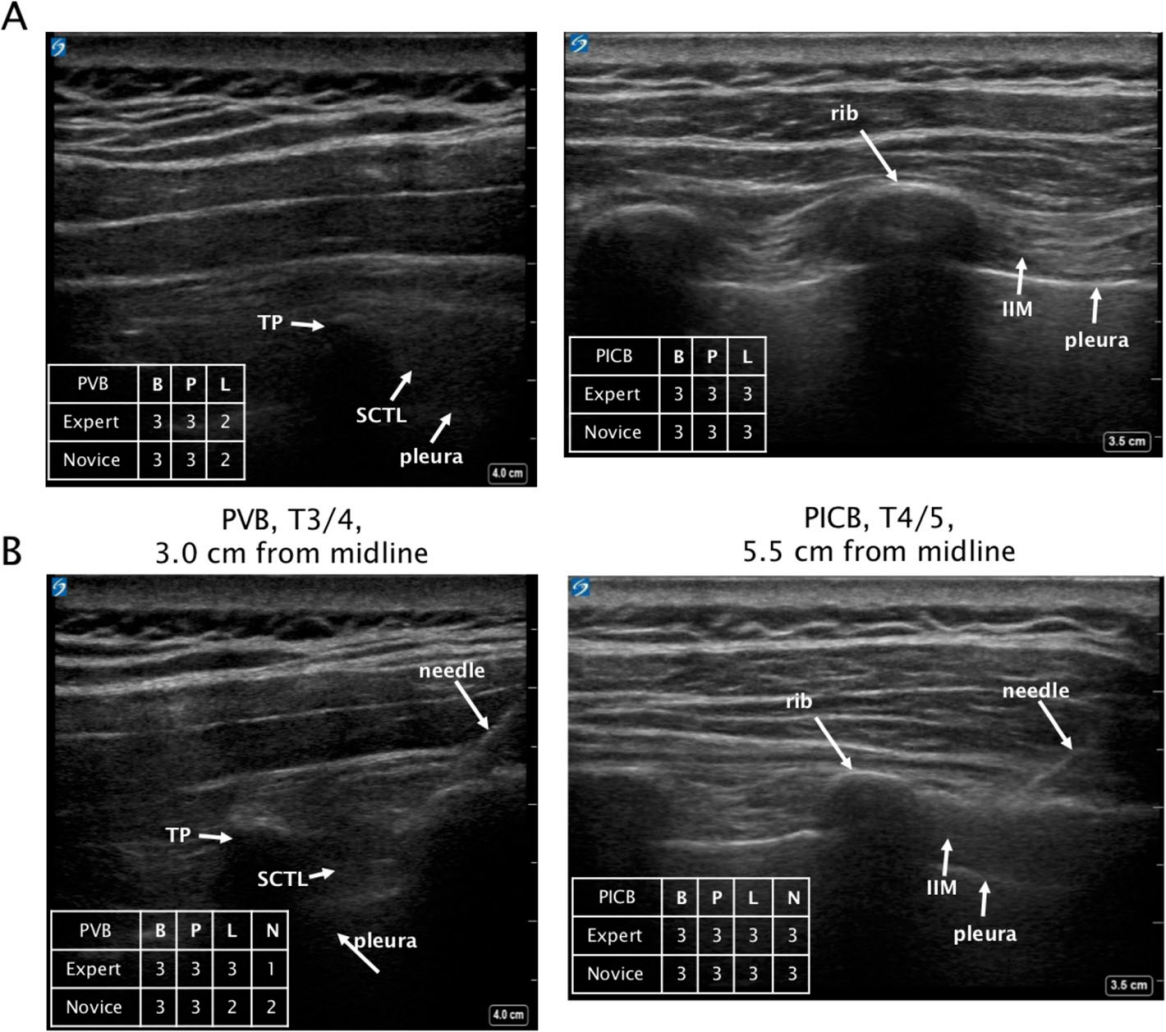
Ultrasound Images Representative highly rated block images before needle insertion for PVB (a) and PICB (b), and immediately before LA administration for PVB (c) and PICB (d). US visualization scores of expert and novice regarding quality of block elements are noted in tables on each image (B:bone, P:pleura, L:ligament/membrane, N:needle). PICB: proximal intercostal block, PVB: paravertebral block, LA: local anesthetic
